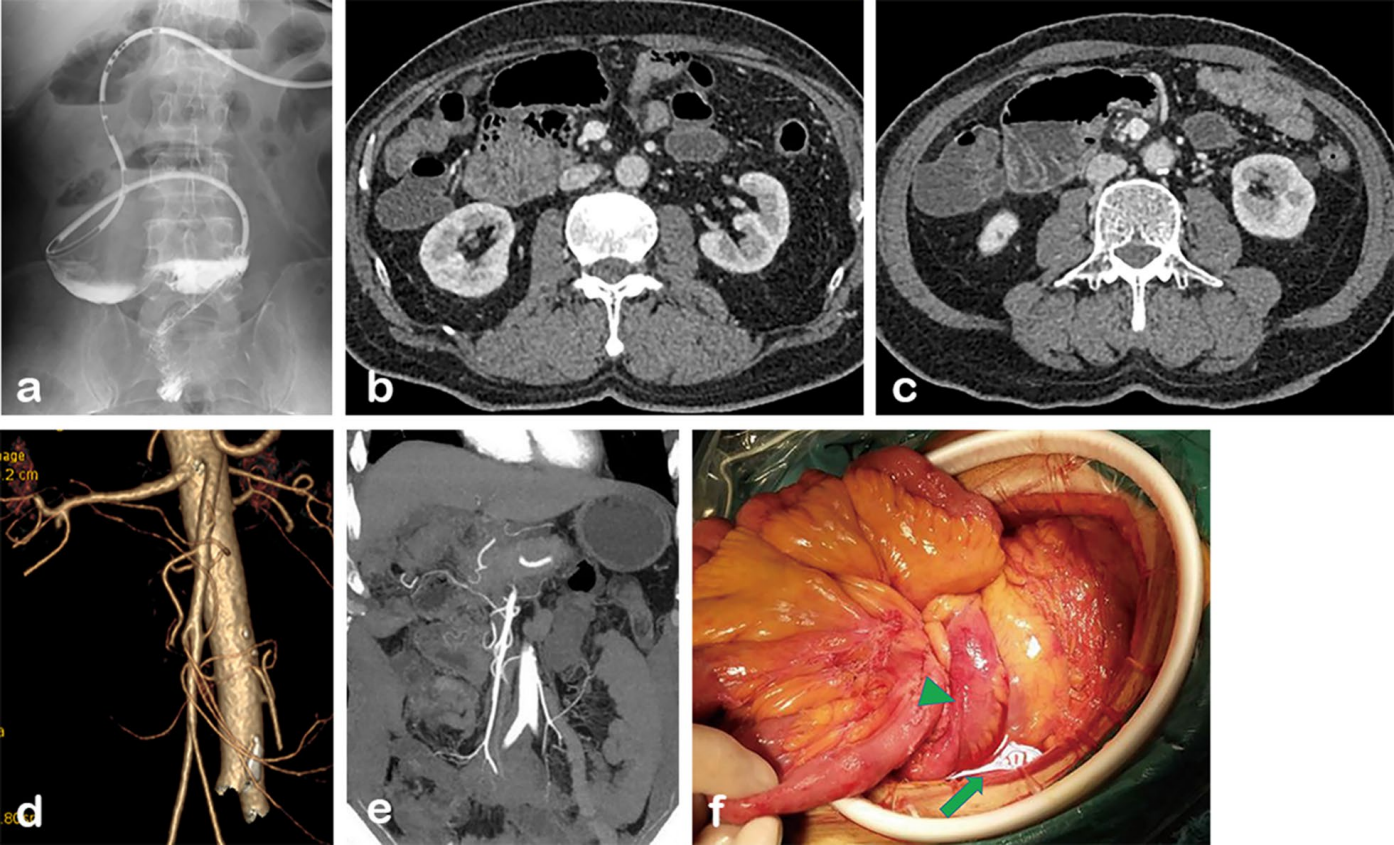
A 64-year-old man developed volvulus after radical resection of rectal cancer (D_N_J_R_C_R_, type 1a). a Endoscopic catheterization: After passing through the duodenum, which was located to the right of the midline, the catheter was unable to pass smoothly into the misplaced obstructed proximal jejunum. b, c CT showed that the duodenum and jejunum were located in the right upper abdomen. d, e CTA shows SMA and its branches. 6f: Ladd’s bands were found during surgery (arrow). The terminal ileum was visualized (arrowhead)
